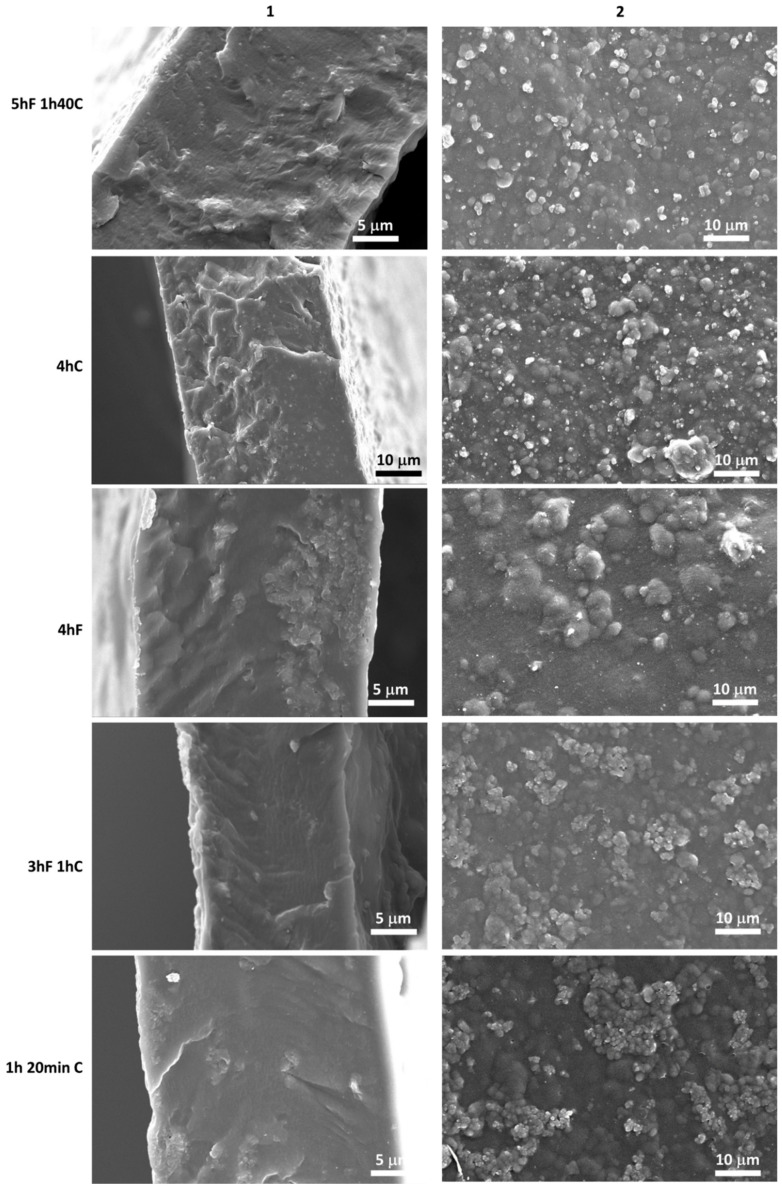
SEM images of cross-section (1) and surface (2) of MMMs at different ultrasound treatments.
